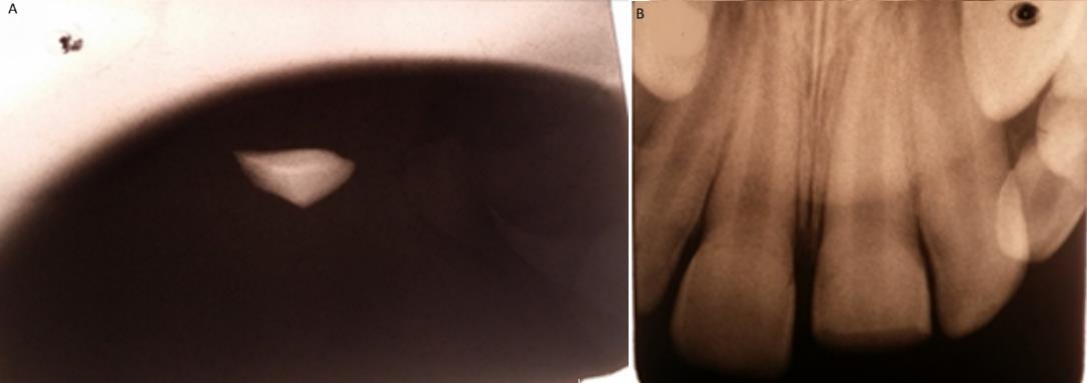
A) radiograph of the lower lip showing the radiopaque tooth fragment; B) radiograph of the central incisor
